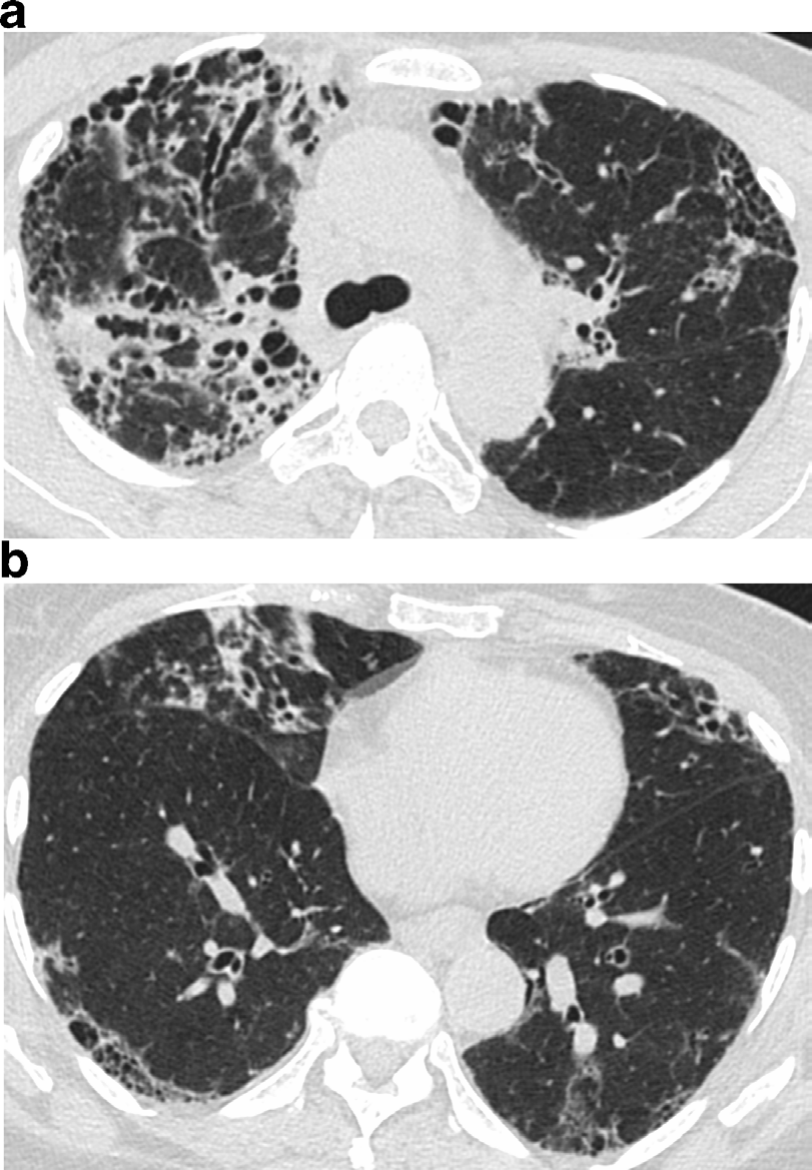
HRCT features suggestive of an alternative diagnosis. There is worse fibrosis on the cranial image (a) compared to a more caudal level (b), with traction bronchiectasis and distortion showing subpleural and bronchovascular distribution. The upper lung predominance suggests a non-IPF diagnosis. This patient was diagnosed with sarcoidosis. HRCT, high-resolution CT; IPF, idiopathic pulmonary fibrosis.
